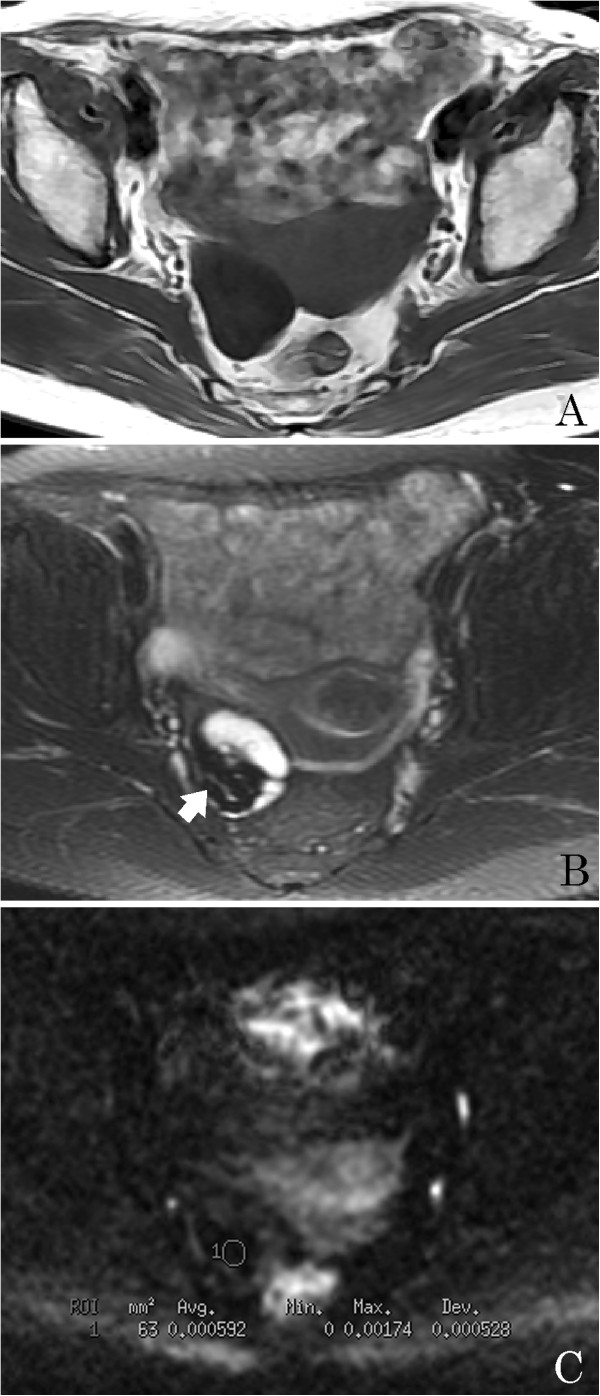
A 67-year-old woman with an ovarian cystadenofibroma. (A) Axial T1-weighted image shows a right ovarian mass with a hypointensity. (B) Axial T2-weighted image reveals that the solid part of the mass is hypointense (arrow) and the cystic component is hyperintense. (C) Axial diffusion-weighted imaging (DWI) reveals that the solid part of the mass has a very low signal intensity (circle 1, apparent diffusion coefficient (ADC) = 0.592 × 10^-3^ mm^2^/s).
